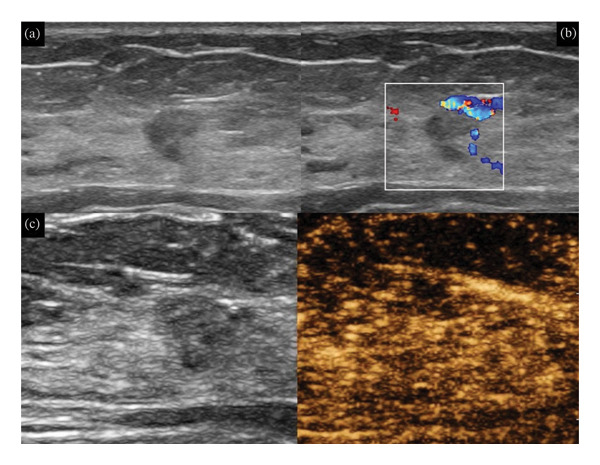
A 28‐year‐old lady with a BI‐RADS 4A lesion in the right breast. The lesion was detected in routine screening without any associated symptom. The maximal diameter of the lesion was 9 mm. In combination with contrast‐enhanced ultrasound, the lesion was downgraded to BI‐RADS 3. Ultrasound‐guided vacuum‐assisted excision was performed. The pathology was adenosis. (a) B‐mode ultrasound showed a hypoechoic lesion with nonparallel orientation and (b) color Doppler detected vessels in rim, and (c) CEUS showed iso‐ and synchronous enhancement with surrounding breast tissue.
